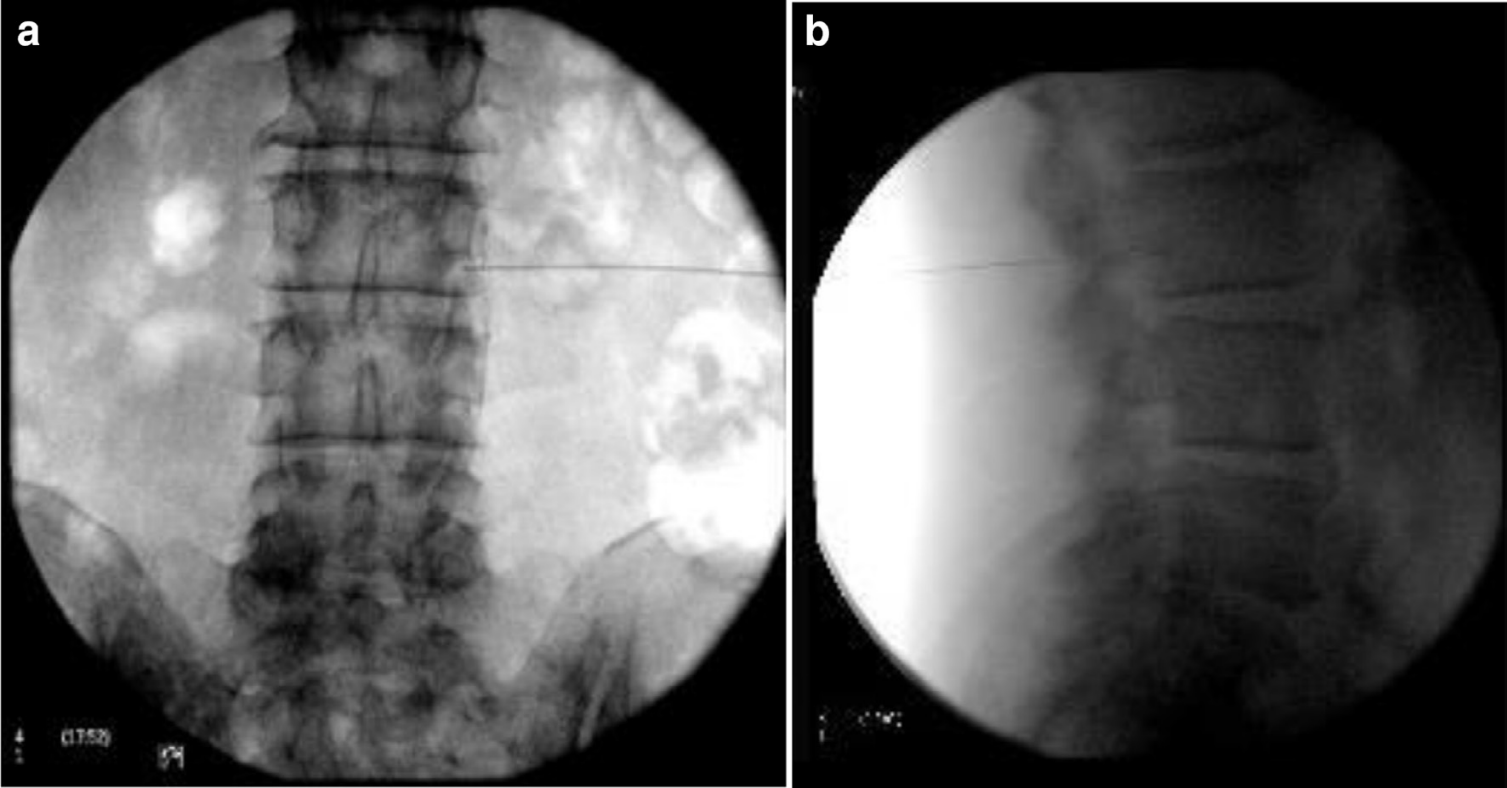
Repeat L3 Nerve root block using C-arm X-ray image in theatres. (a) Coronal image C-arm. (b) Sagittal image C-arm. Figure 4 shows repeat L3 Nerve root block images from C-Arm. Level was localized in Coronal (a) and Sagittal images (b) before giving injection.
